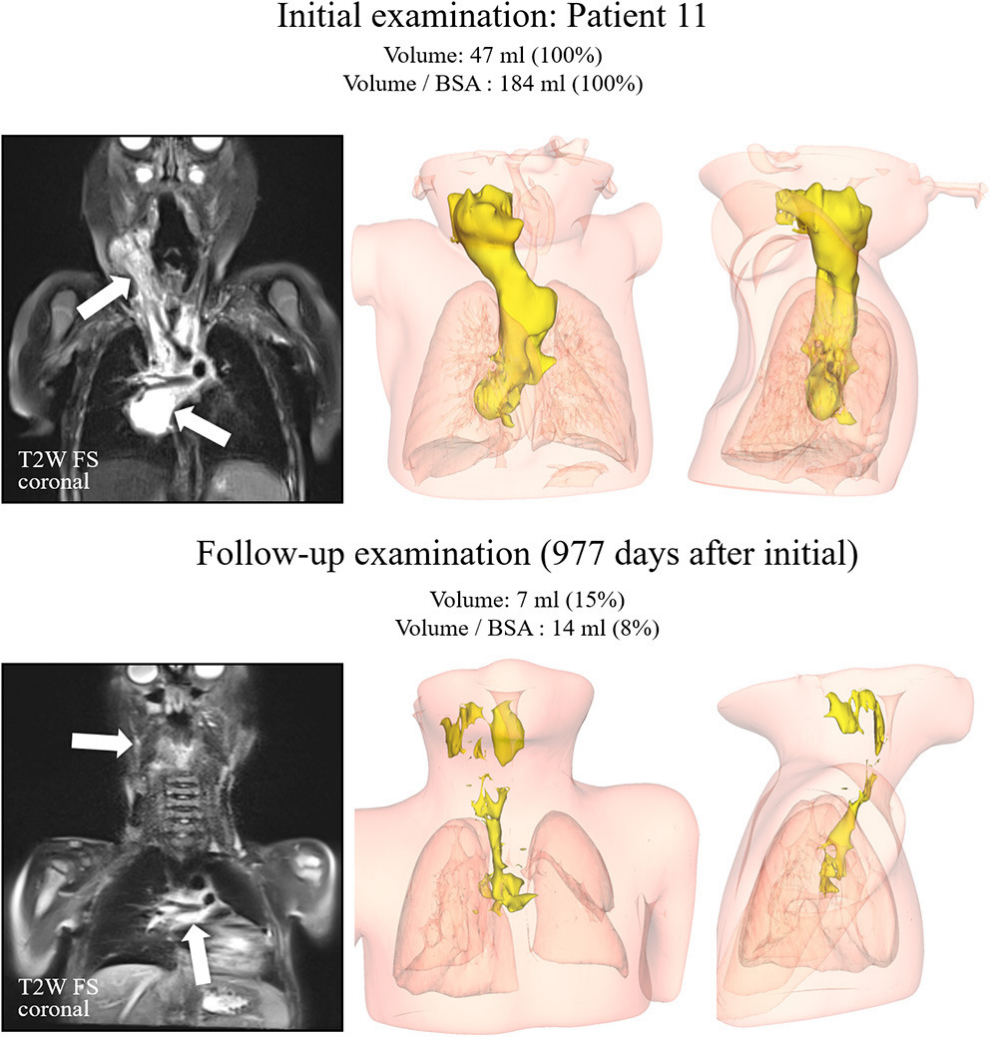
Patient #11. T2-weighted images with fat saturation in the coronal plane on the left side for both examinations. The kaposiform hemangioendothelioma demonstrated a high signal intensity (arrows) on both examinations, with substantial volume reduction over time. Corresponding 3D reconstructions in anteroposterior and mediolateral views are displayed in the middle and right columns.
